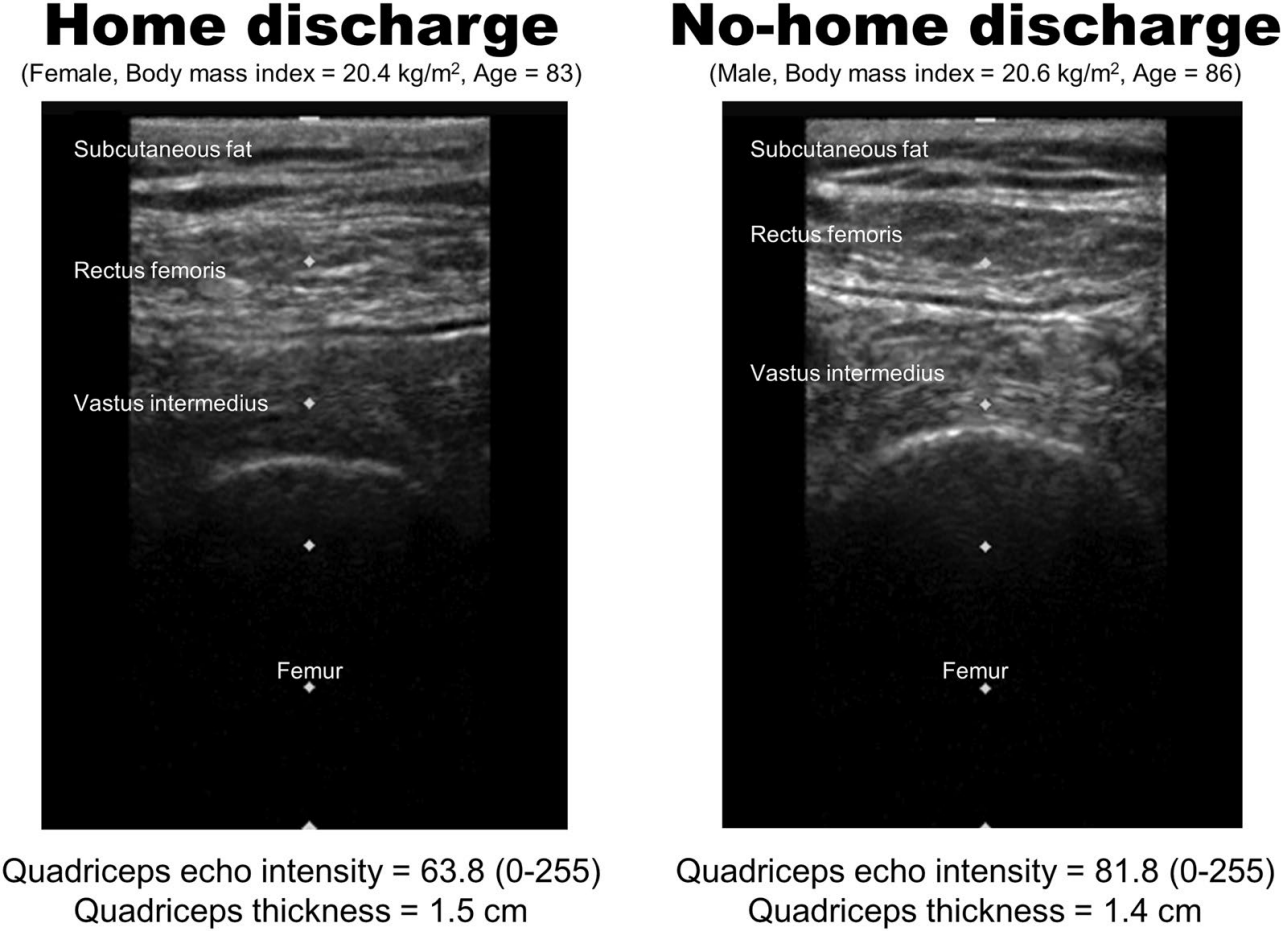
Typical ultrasound images of patients with home discharge and no-home discharge.
